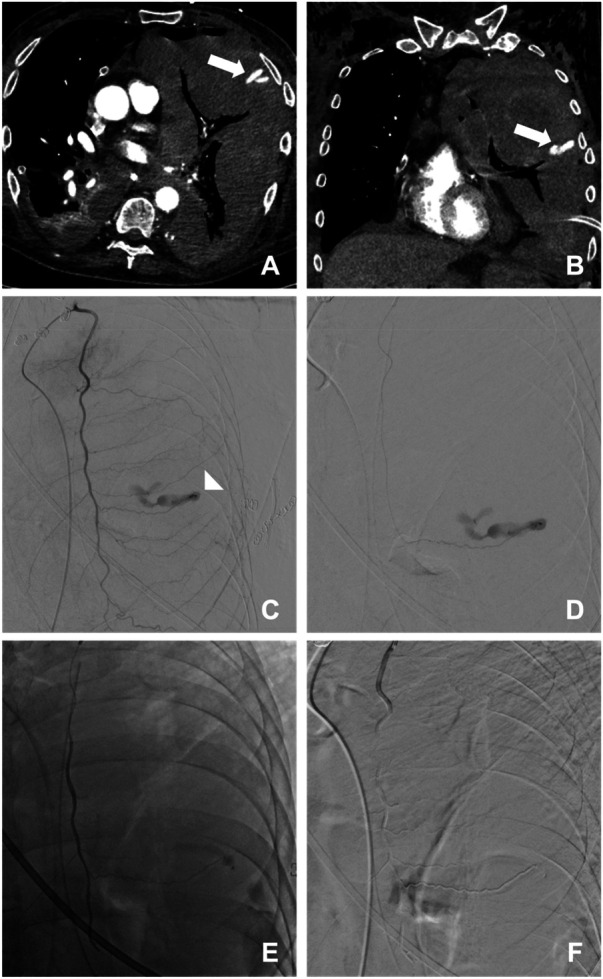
A 68-year-old man with sequelae of polytrauma. (A,B) Axial and coronal contrast-enhanced CT images demonstrate active contrast extravasation from the left internal thoracic artery secondary to a rib fracture (bold arrows). (C) Selective angiography confirms active bleeding arising from an anterior intercostal branch (arrowhead). (D) Superselective catheterization of the bleeding vessel is performed. (E) Embolization is achieved using n-butyl cyanoacrylate mixed with ethiodized oil in a 1:2 ratio. (F) Final angiographic control demonstrates complete and successful embolization of the internal thoracic artery with no evidence of residual bleeding.
